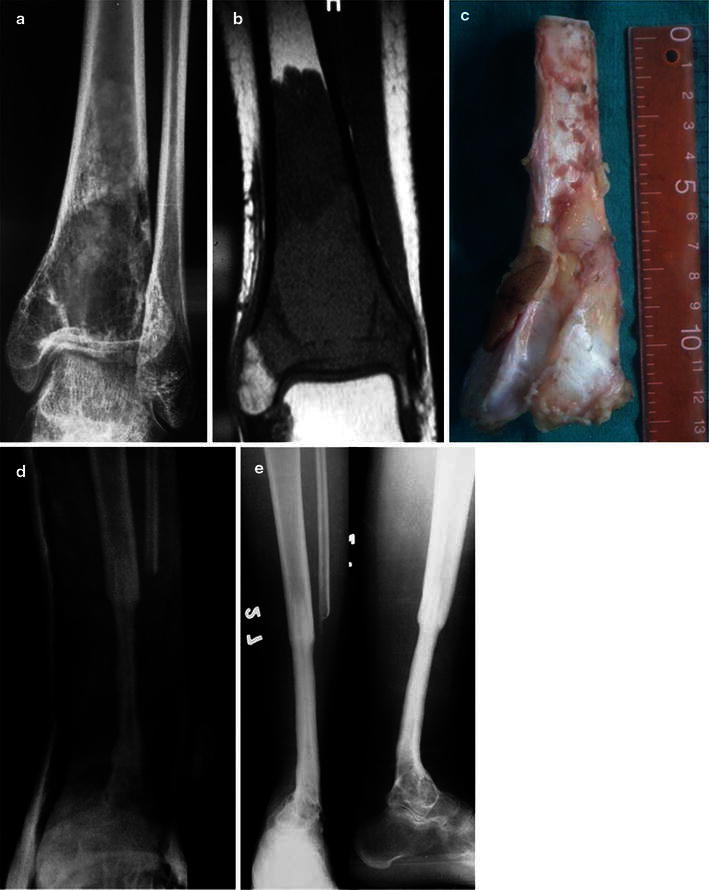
a Radiograph showing osteosarcoma of the distal tibia in a 22-year-old male, b MRI of the same patient, c resected distal tibia, d X-ray at 8.5 months follow-up, e X-rays at 54 months follow-up showing hypertrophy of the fibula
